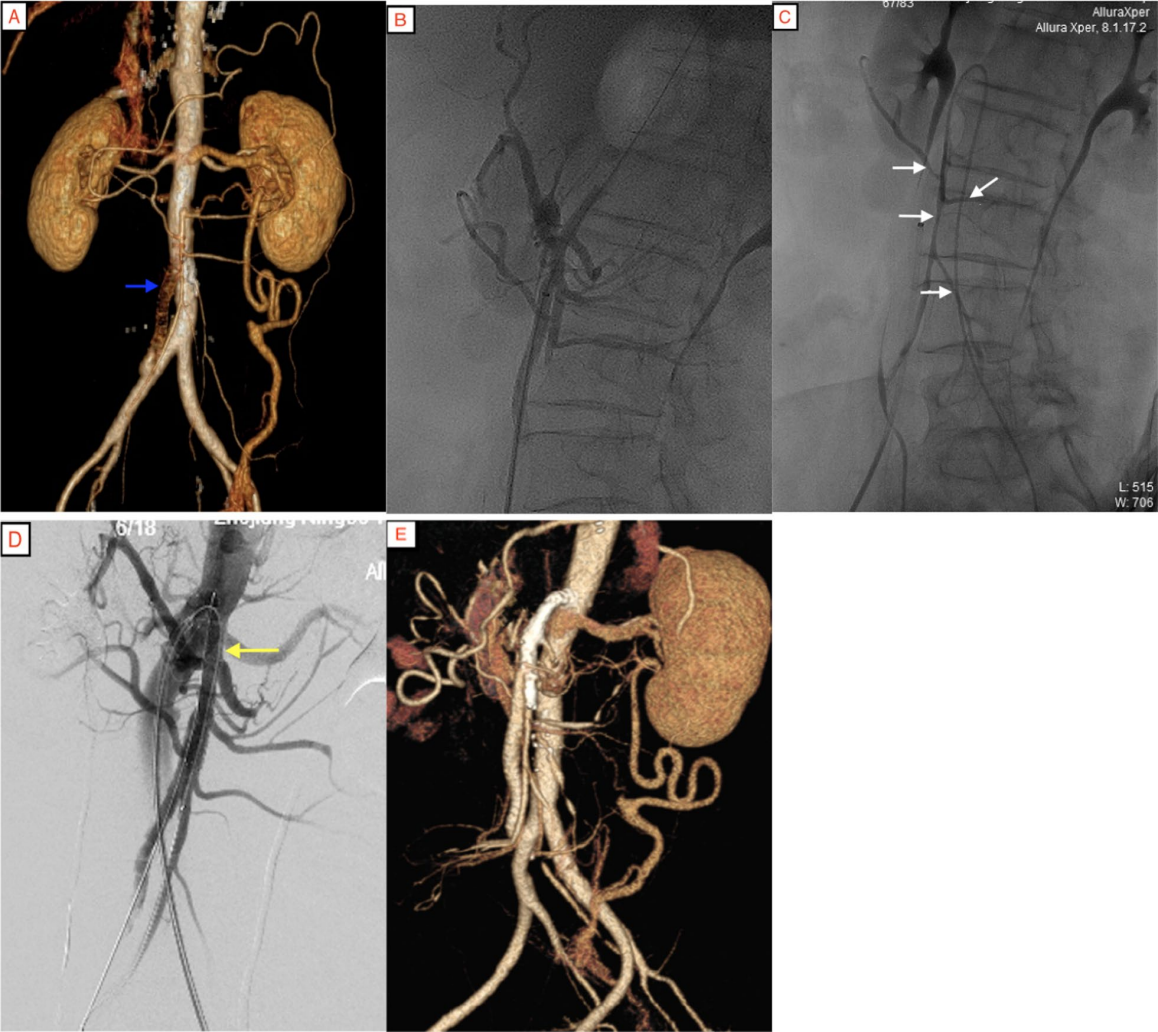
A Artificial vessel occlusion (blue arrow), considering thrombosis. B The guidewire passes through the occluded SMA segment to the abdominal aorta. C SMA and branch development (white arrow) are seen in anterograde angiography through left femoral artery catheterization. D The blood flow returns smoothly after SMA stent (yellow arrow) is placed. E CTA reexamination after surgery
